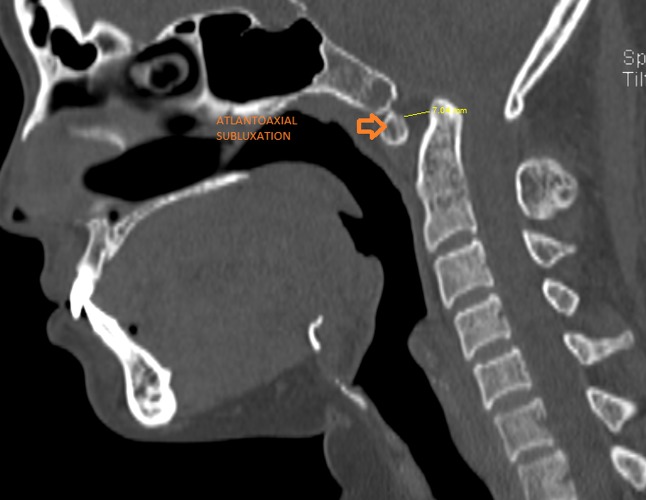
Sagittal computed tomogram showing increased atlanto-dens interval (7 mm) suggestive of atlanto-axial instability (arrow)
